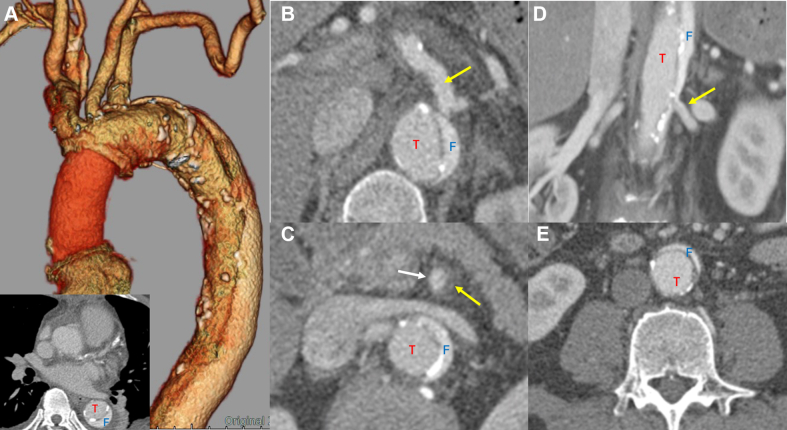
Postoperative Computed Tomography Angiography (A) Three-dimensional reconstruction demonstrating graft replacement of the ascending aorta and axial image at the level of the mid ascending aorta. (B) Stable anatomy of the celiac artery (arrow). (C) Re-expansion of the superior mesenteric artery true lumen (white arrow) with regression of the false lumen (yellow arrow). (D) Persistent perfusion of the left renal artery without new complications (arrow). (E) Dilated true lumen and regressed false lumen at the level of the distal abdominal aorta. F = false lumen; T = true lumen.
